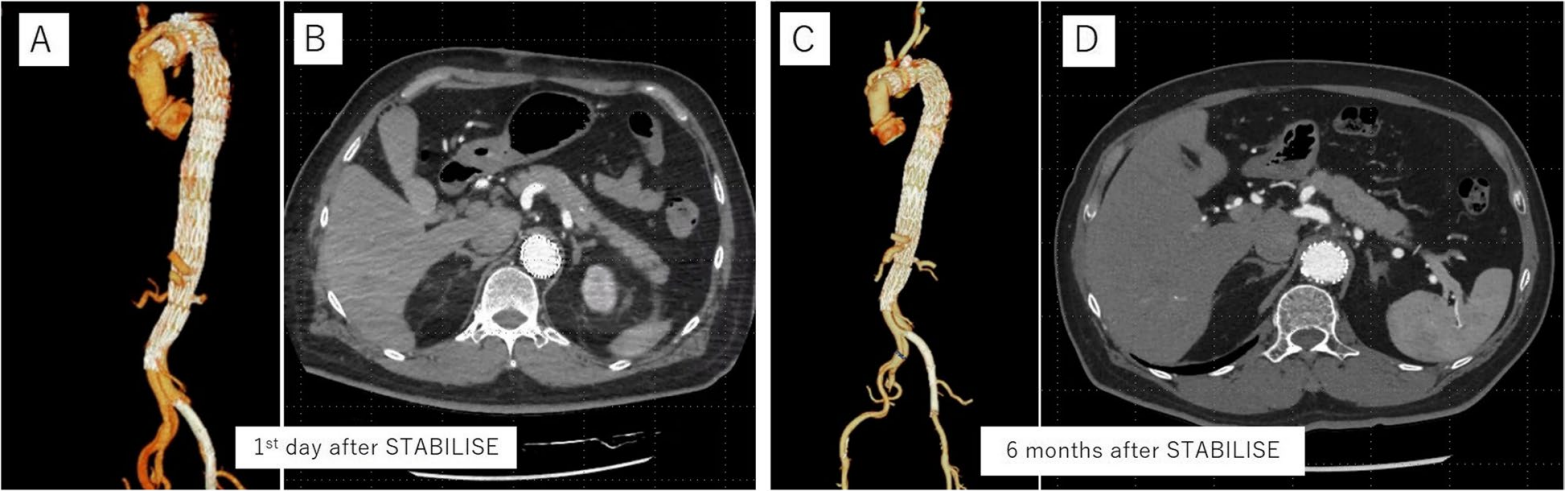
A–D CT images after STABILISE technique. Legend: A and B. 3D image (A) and axial image (B) of the CT scan at the 1st day after STABILISE technique. False lumen of the aorta was obliterated where STABILSE technique was applied. C and D. 3D image (C) and axial image (D) of the CT scan at 6 months after STABILISE technique. Good aortic remodeling was maintained without dilation of aorta
